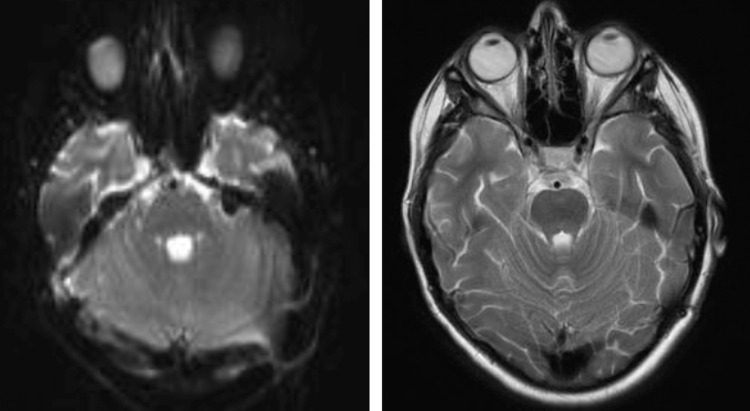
Postoperative showed total tumor resection in FLAIR (left) and MRI T2 (right). FLAIR: fluid-attenuated inversion recovery
